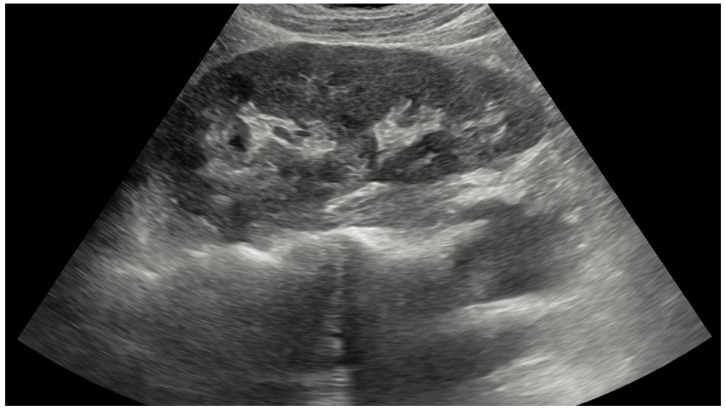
Ultrasound image of normal kidney with centrally located hyperechoic renal sinus surrounded by hypoechoic renal parenchyma.
